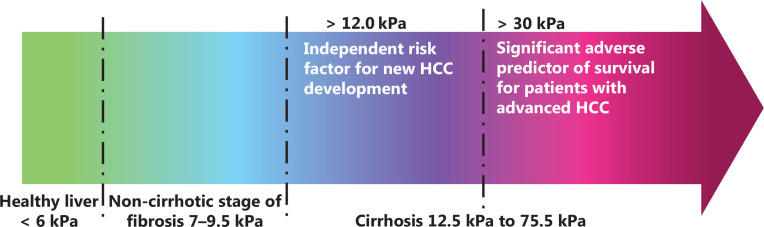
Pathological changes in liver tissue stiffness, detected by ultrasound transient elastography. The tissue stiffness increases from normal liver tissue to liver fibrosis and cirrhosis. The stiffness of normal liver tissue is below 6 kPa^20^. The stiffness of the non-cirrhotic stage of fibrosis (F1–F3) is 7–9.5 kPa^8^. The stiffness of F4 fibrosis (cirrhosis) is > 12.5 kPa^20^. The range of liver cirrhosis stiffness is similar to that of liver cancer tissue. Liver stiffness > 12.0 kPa is an independent risk factor for new HCC development^10^. Liver cancer tissue > 30 kPa is a significant adverse predictor of survival in patients with advanced HCC^21^.
